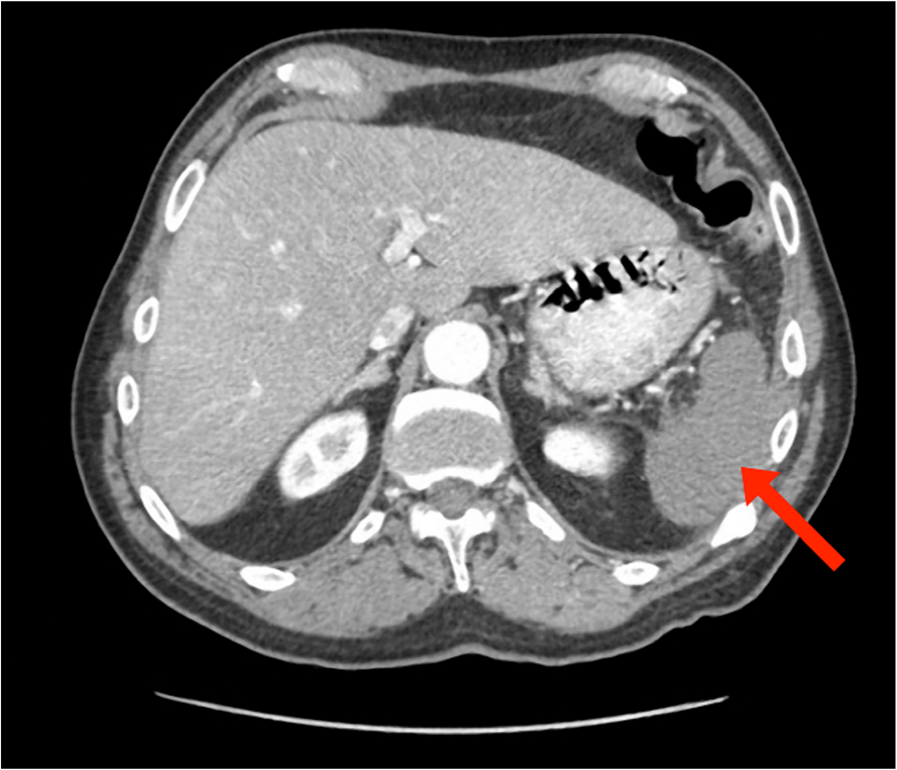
CT-scan of the abdomen obtained on presentation showed complete non-enhancement of the spleen (arrow) supporting complete splenic infarction.
